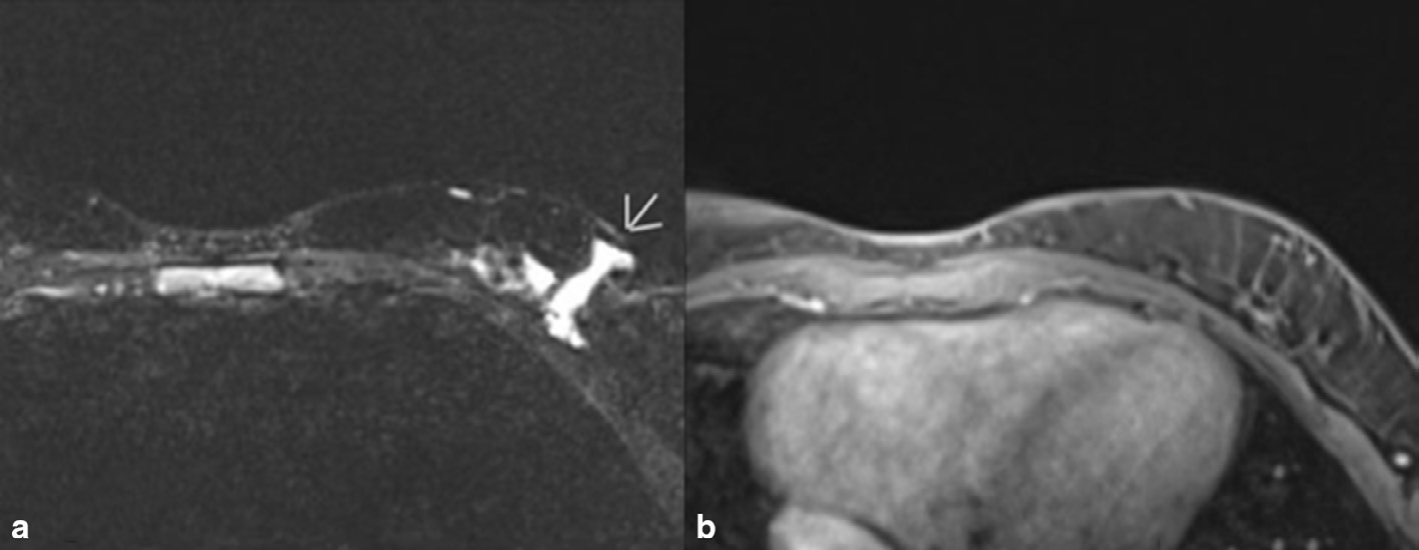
A and B. MRI images of left breast with contrast most recently. (A). Image shows recurrence of lymphatic malformation. Impression was overall benign with 2.6 × 0.5 cm serpinginous area of T2 hyperintense signal in the lower outer quadrant of the left breast with hyperintense T2 signal extending inferolaterally with the distal aspect excluded by collimation. These findings are located at the surgical bed and are suspicious for residual disease. (B). *T_1_* weighted fat-suppressed contrast-enhanced sequence no significant enhancement.
